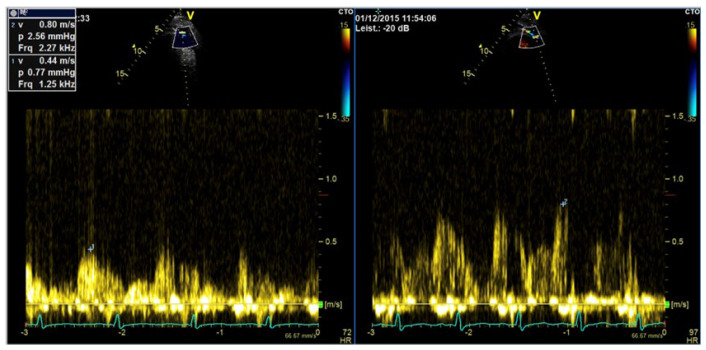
Measurement of coronary flow velocity reserve with contrast-enhanced PW Doppler; (left) diastolic flow velocity at rest; (right) diastolic flow velocity at maximum vasodilation with adenosine (170 micrograms/kg body weight). Flow velocity reserve 1.8, indicating stenosis of the LAD with or without microvascular dysfunction.
